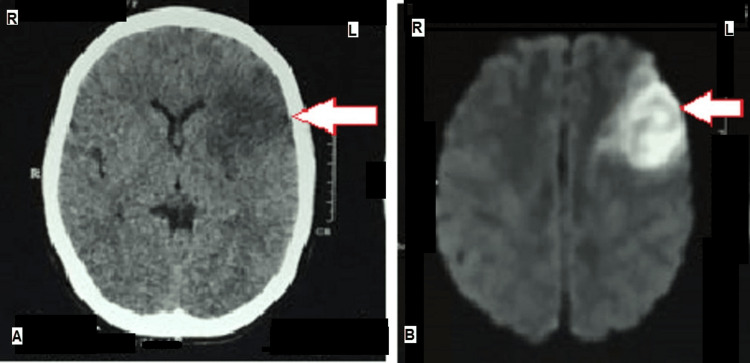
(A) NCCT brain showing left-sided cortical hypodensity (arrow) corresponding to acute infarct. (B) DW-MRI of the brain showing diffusion restriction (hyperintensity) in the left cerebral cortex (arrow) and left gangliocapsular region suggestive of acute non-hemorrhagic infarct. R- Right, L- Left, NCCT- Non-contrast computed tomography, DW-MRI: Diffusion weighted-magnetic resonance imaging
